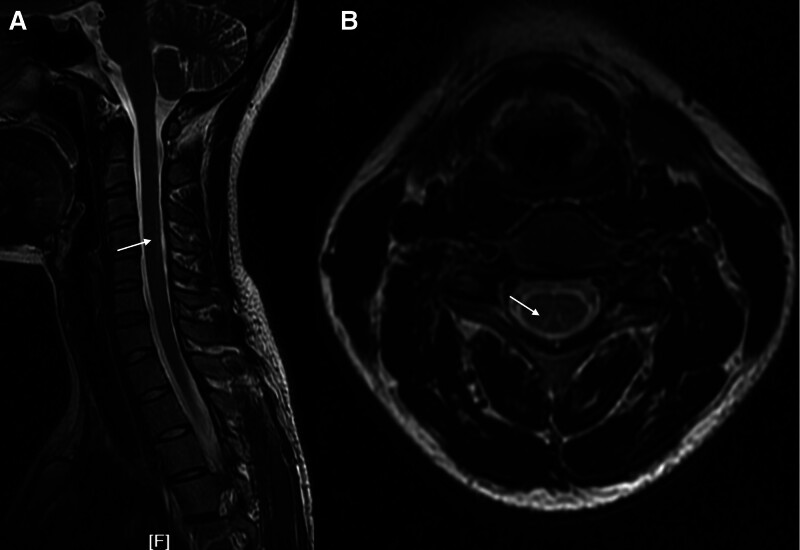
MRI of the cervical spine. The T2 sagittal image (A) shows dorsal column T2 hyperintensity extending over segments C3-C5 (white arrow) and the axial image (B) shows an “inverted V sign” (white arrow). MRI = magnetic resonance imaging.
